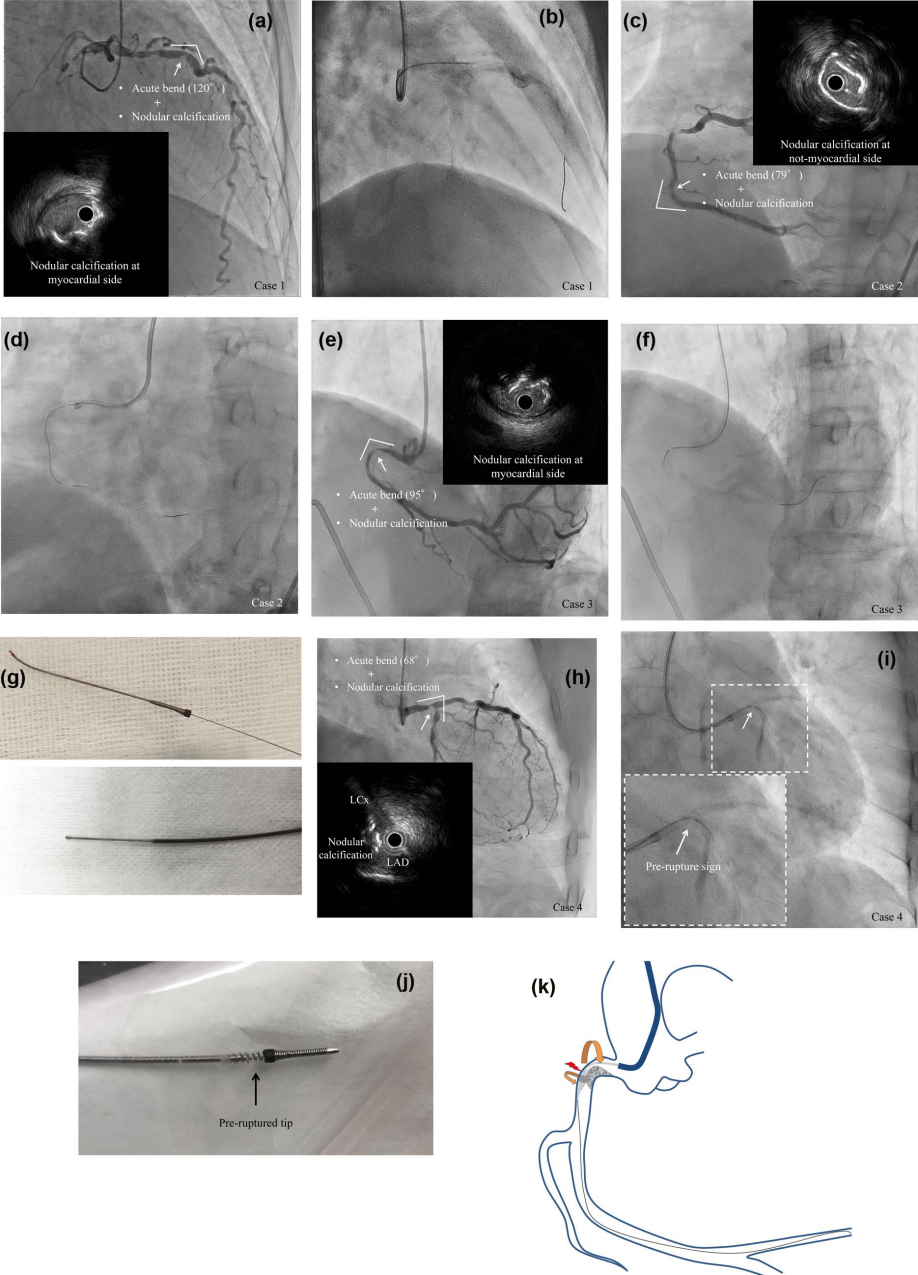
OAS tip rupture cases and a speculation of this phenomenon. a Initial angiogram and IVUS image of case 1 (white arrow points to nodular calcification). b Rupture of OAS tip (Case 1). c Initial angiogram and IVUS image of case 2. d Rupture of OAS tip (Case 2). e Initial angiogram and IVUS image of case 3. f Rupture of OAS tip (Case 3). g Ruptured tip of OAS and its shaft. h. Initial angiogram and IVUS image of case 4. i Radiolucent band reveals pre-ruptured tip of OAS. j Pre-rupture of OAS tip (Case 4). k Speculation over causes of OAS tip rupture. IVUS, intravascular ultrasound; OAS, orbital atherectomy system
